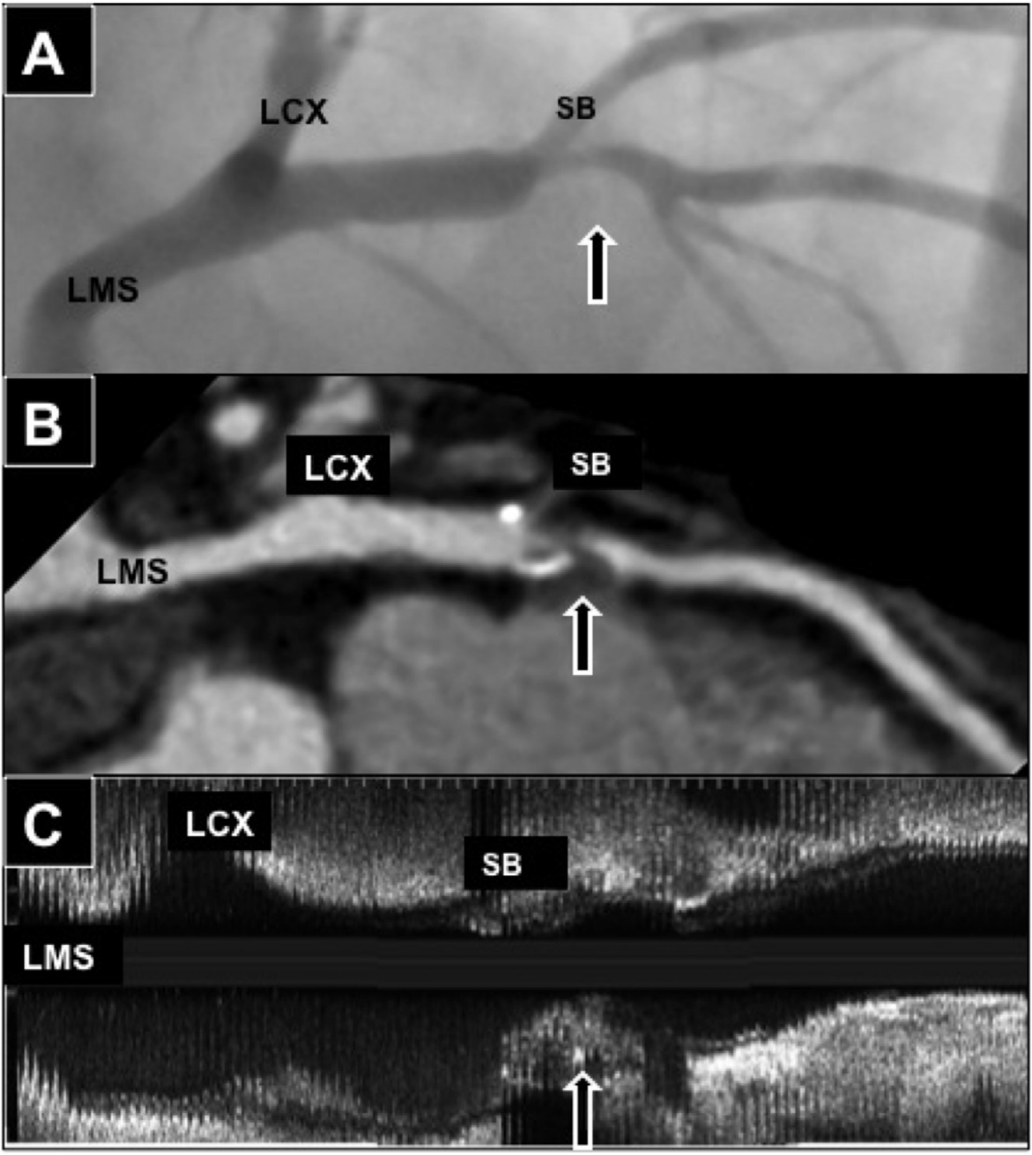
Co-registration of CT, IVUS and angiography. Fibroatheroma plaque with large necrotic core in Left anterior descending coronary (Arrow) in (A) Catheter angiography (B) CT curved multi-planar reformat and (C) IVUS longitudinal view. Co-registration performed using distances from Left main stem (LMS), LCX-left circumflex artery and SB-side branch.
